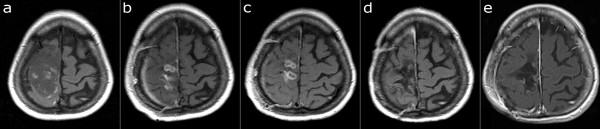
Serial MR imaging in Case 1. T1-weighted image after application of contrast agent. a) April 2004, before surgery. GBM located in the right central region. b) July 2004, post-operative radiotherapy and chemotherapy. Two enhancing lesions present. c) September 2004, one month after the start of TTField therapy. The dorsal enhancing lesion increased in size, highly suspicious of tumor recurrence. d) June 2005, TTField treatment. No enhancing lesion present. e) August 2011. No enhancing lesion present. GBM, glioblastoma multiforme; MR, magnetic resonance; TTField, tumor-treating fields.
